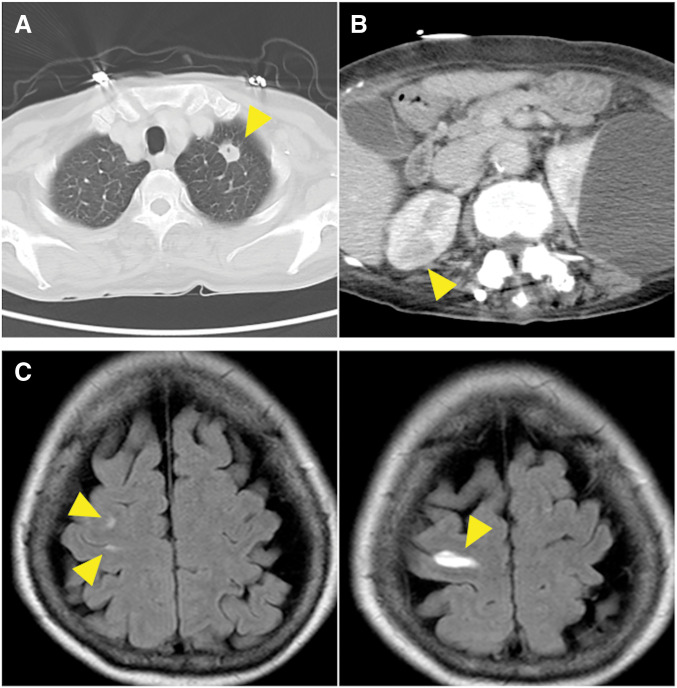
Preoperative images obtained with CT and MRI. CT images showing multiple embolisms in the lungs and kidneys (yellow arrowheads). Embolisms in the lungs with suspected derivation via the patent ductus arteriosus (A and B). Microbleeds attributable to vegetations are observed in the brain (yellow arrowheads) (C).
